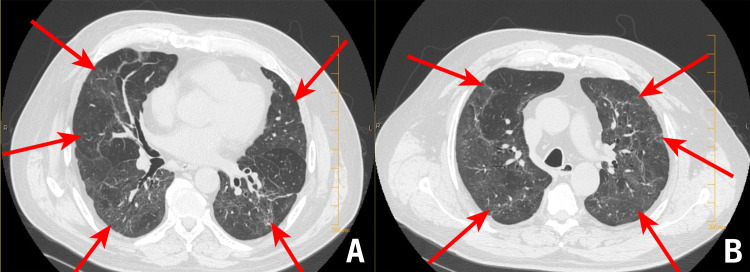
Follow-up CT scan performed six weeks after discharge A and B: images from the follow-up CT scan demonstrating significant resolution of the fibrotic changes present at the time of admission CT: computed tomography
